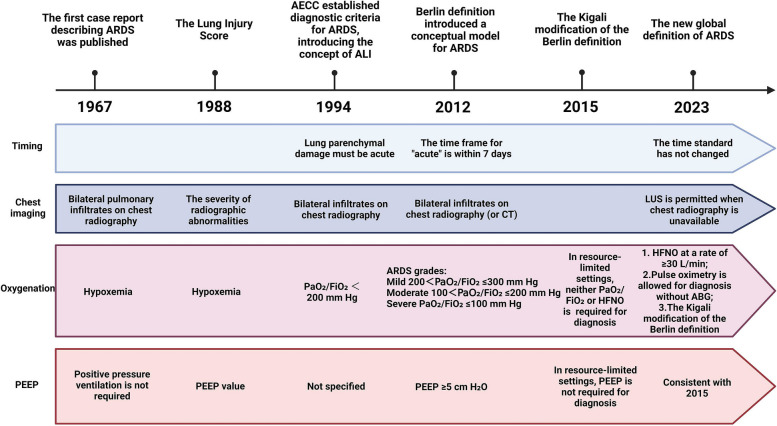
Evolution of the diagnostic criteria for acute respiratory distress syndrome (ARDS). This figure outlines key milestones in the evolution of ARDS diagnostic criteria across four domains: time, chest imaging, oxygenation, and positive end-expiratory pressure (PEEP). AECC: American-European Consensus Conference; ALI: Acute lung injury; CT: Computed tomography; LUS: Lung ultrasound; PaO₂: Partial pressure of arterial oxygen; FiO₂: Fraction of inspired oxygen; HFNO: High-flow nasal oxygen; ABG: Arterial blood gas; PEEP: Positive end-expiratory pressure
